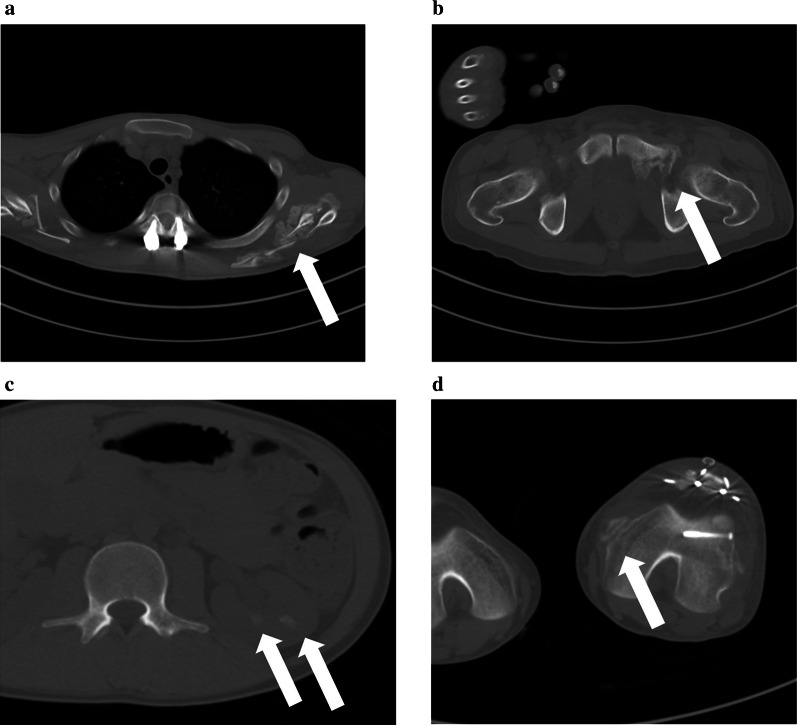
Heterotopic ossifications on computed tomography. Heterotopic ossifications are observed in the scapula (a), pubis (b), tibia (c), and renal calculus (d; white arrows)
